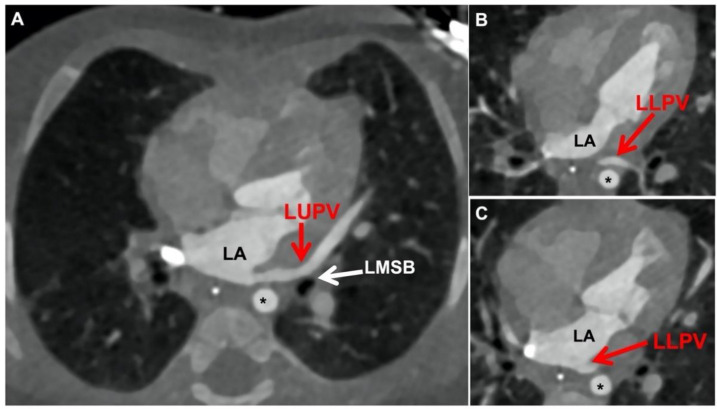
Left pulmonary veins (CT images). (A) Left upper pulmonary vein takes long, angulated course as it passes from lung parenchyma over left mainstem bronchus, across pericardial reflection into left atrium medially; (B) Left lower pulmonary vein courses anteriorly over the descending thoracic aorta taking an angulated course; (C) Left lower pulmonary vein crossing the pericardial reflection and entering far medially into left atrium. (LA = left atrium; LUPV = left upper pulmonary vein; LMSB = left mainstem bronchus; LLPV = left lower pulmonary vein; Asterisk = descending thoracic aorta).
